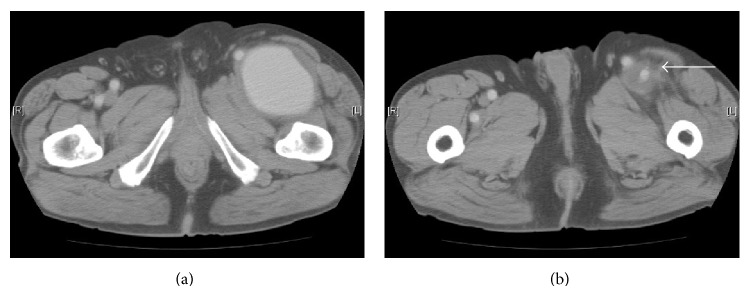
Computed tomography showed a 78 × 86 mm left profunda femoris artery (PFA) (a), which extended to the distal part of the left PFA (white arrow) (b).
